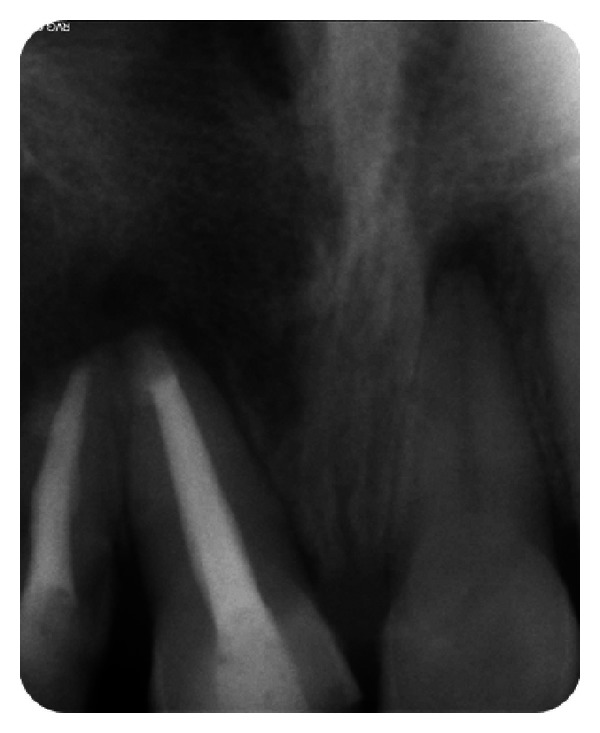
18-month followup IOPA radiograph.
